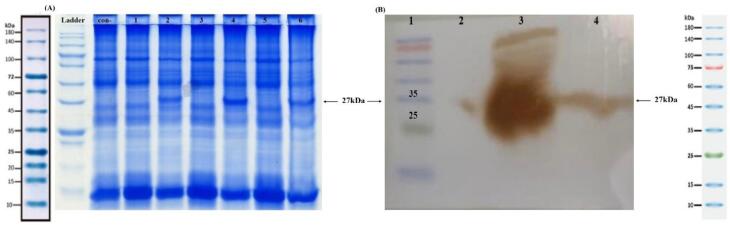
###  Purification of PSMA-Nb protein and western blotting
